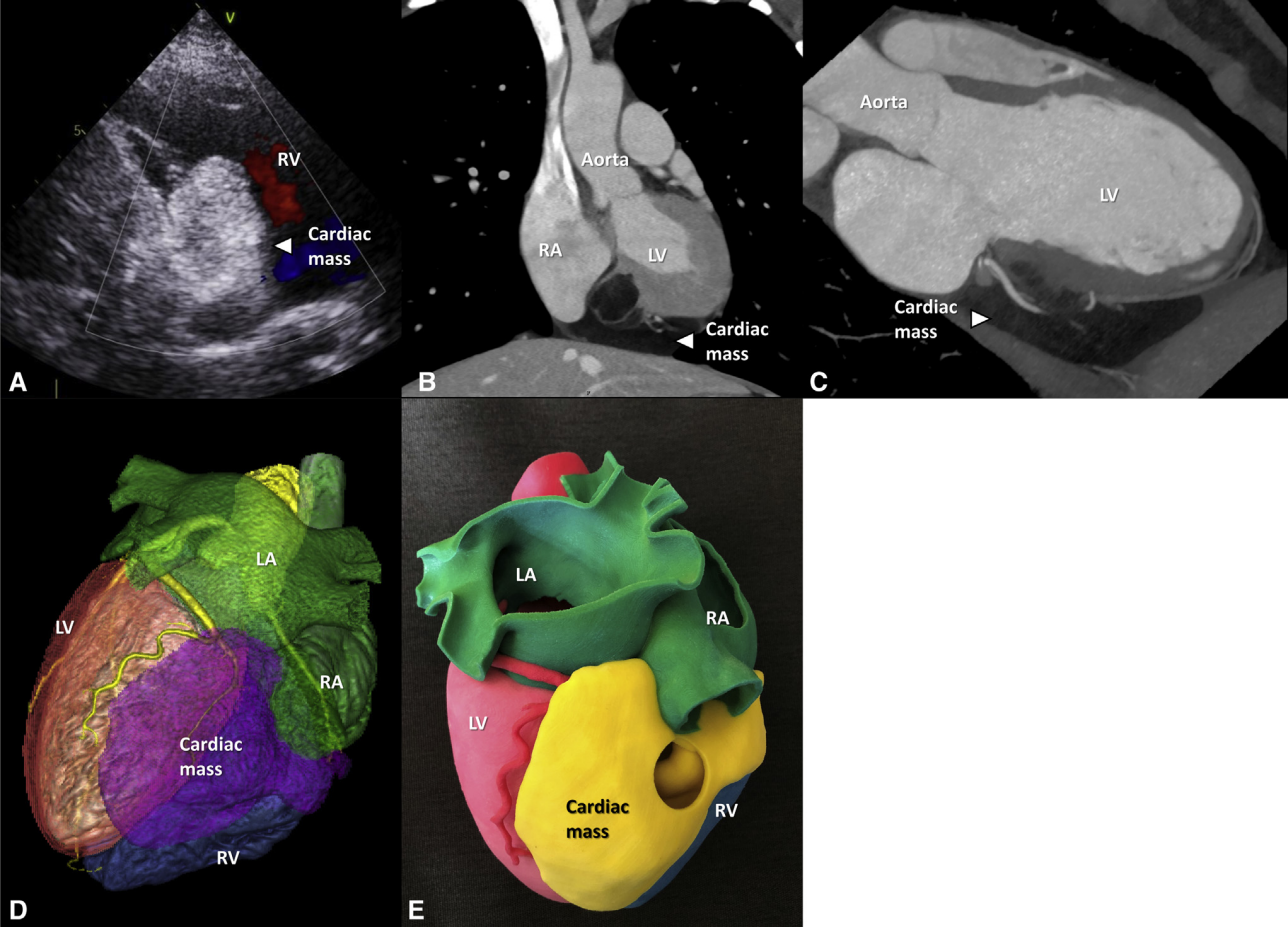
Preoperative image findings of cardiac tumor. A, Transthoracic echocardiographic image. B-D, Computed tomography image of the heart showing a cardiac mass measuring 66 mm × 70 mm × 40 mm. E, Three-dimensional printing model. *RV*, Right ventricle; *RA*, right atrium; *LV*, left ventricle; *LA*, left atrium.
